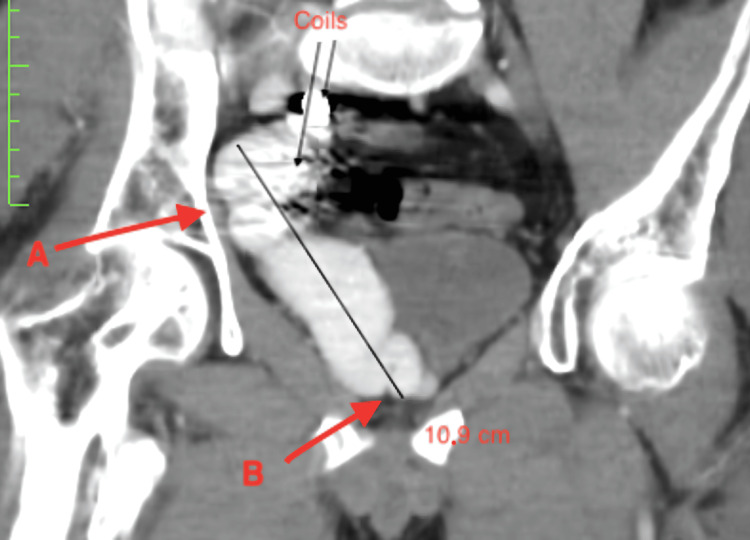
CT angiography. A coronal section of the pelvis (slight rotation of 10° to the right of the vertical axis). Note the coils, the enormous right internal iliac vein (A), and some of its tributaries (B) two years after endovascular therapy. Also, note the displacement of the bladder toward the midline.
